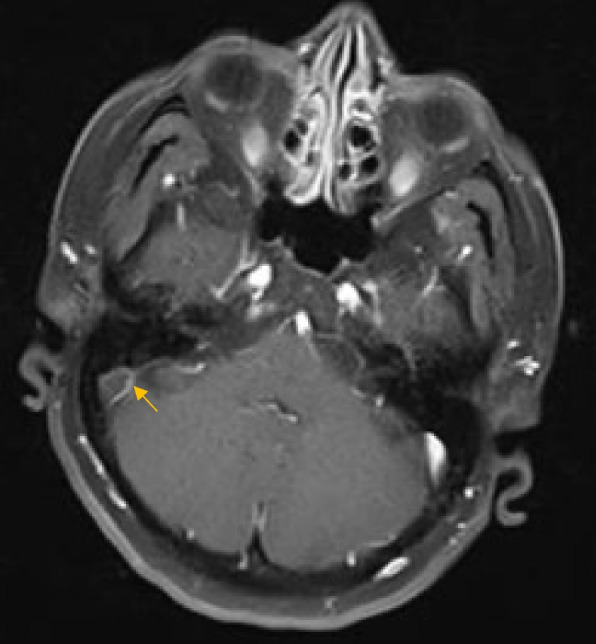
Contrast-enhanced T1-weighted MRI of the brain in axial plane showing thrombosis in the right transverse sinus
